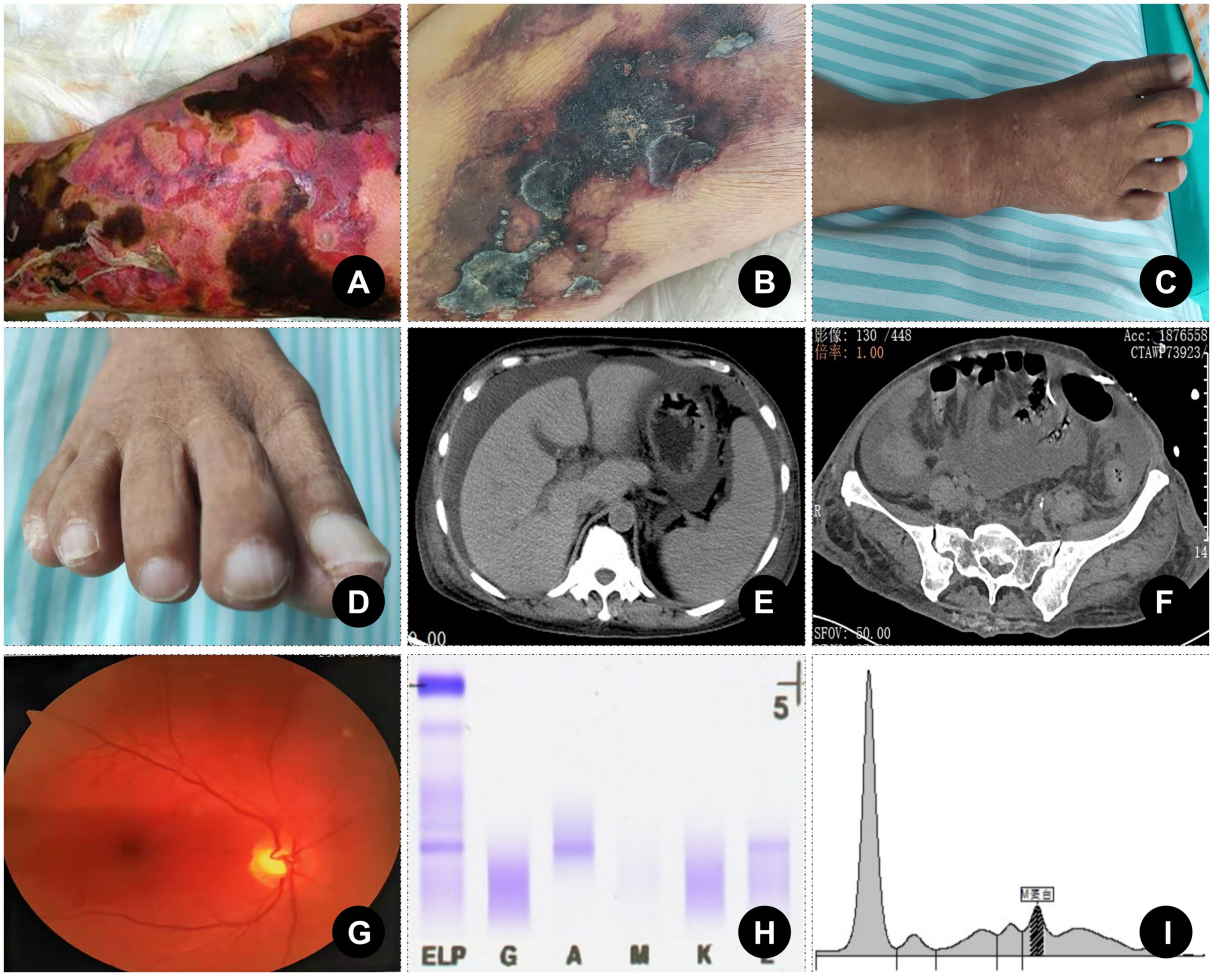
Skin changes and key diagnostic evidence. (A) Skin changes in the lower limbs caused by *Vibrio vulnificus* infection (the past real case at our center with written informed consent obtained). (B) Skin changes in the lower limbs of the case. (C) Pigmentation of lower limb skin and whitening of toenails (distant view). (D) Pigmentation of lower limb skin and whitening of toenails (close view). (E) A cross-sectional CT scan suggested ascites and splenomegaly. (F) Pelvic CT showed irregular sacroiliac joint margins and mild joint space narrowing without lytic lesions. (G) Fundus examination was unremarkable. (H) Elevated serum VEGF (729.71 pg./mL, reference range: 9–86 pg./mL). (I) Monoclonal IgA-λ protein on serum immunofixation electrophoresis.
